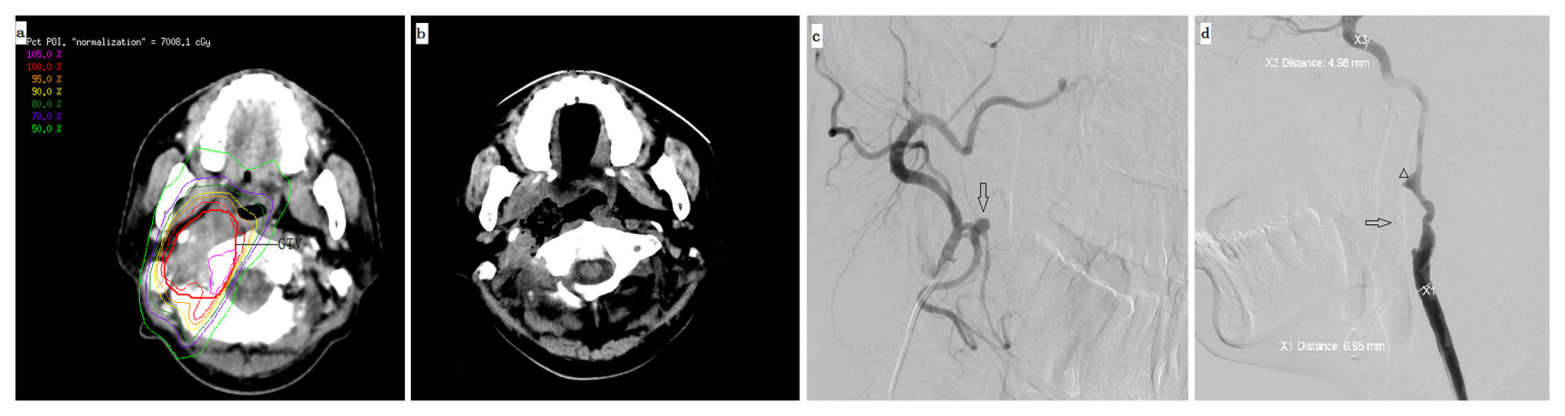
One patient who had haemorraghe after reirradiation: (a) showed the treatment plan for a patient with recurrent foci invading the poststyloid space and so on, (b) showed the CT scan four months after IMRT of the necrosis of nasopharyngeal mucose, and a cavity continuing from the pharynx was formed after the tissue was expelled, (c) the patient had several attack of haemorraghe, DSA showed the right lingual artery pseudoaneurysm (arrow) five months after IMRT, (d) the patient again had massive haemorraghe six months after IMRT, DSA showed pseudoaneurysm in right internal carotid artery (arrow head), coil (arrow) placed at the lingual artery level for (c).
